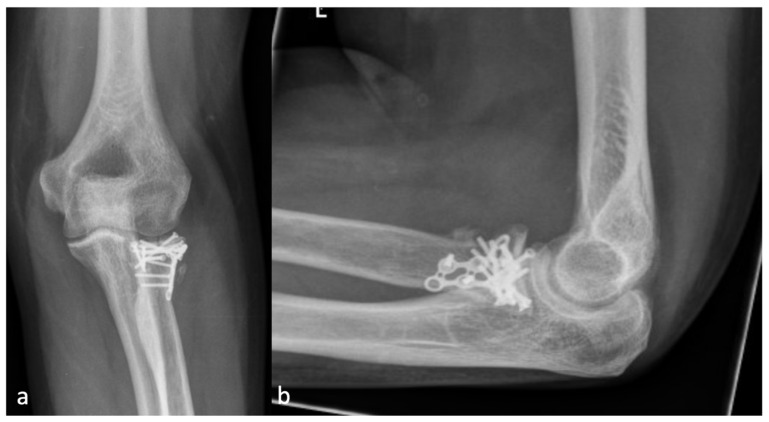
(a) The AP and (b) the lateral X-ray with a radial HO smaller than the diameter of the radial head, which represents an HO 1 r.
